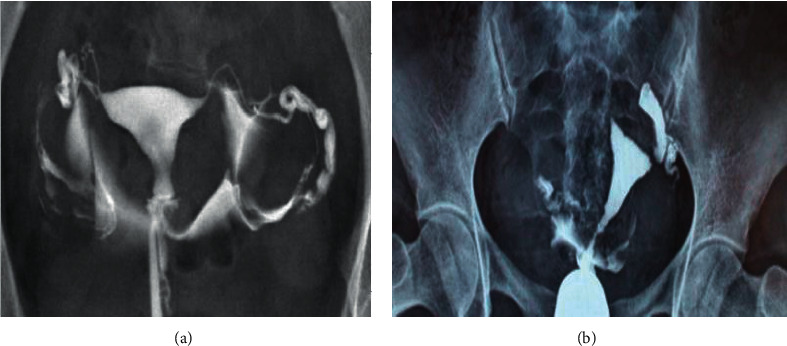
Results of routine MRI examination. (a) The obstructed left fallopian tube; (b) the poorly obstructed left fallopian tube.
